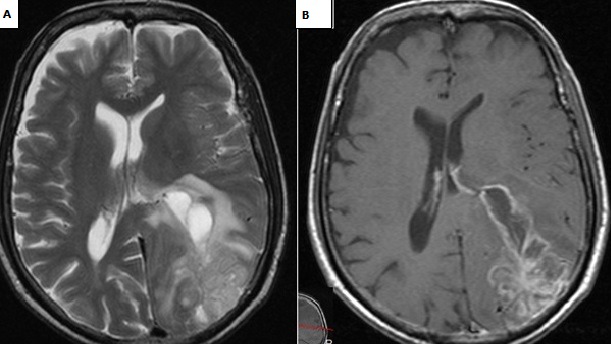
MRI Brain: A) axial; T2W1 B) T1W1 show heterogeneous peripheral left parieto-occipital solid mass with a cystic component; heterogeneous enhancement with rim enhancement seen in B) in keeping with high grade malignancy
